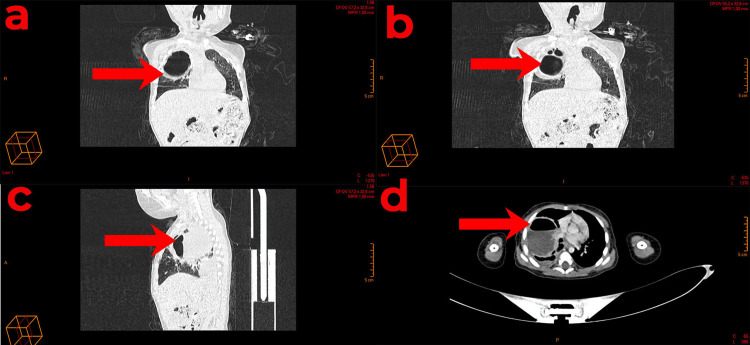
A computed tomography (CT) scan displaying a lung abscess (A, B) Coronal views, (C) a sagittal view, (D) a transversal view.
